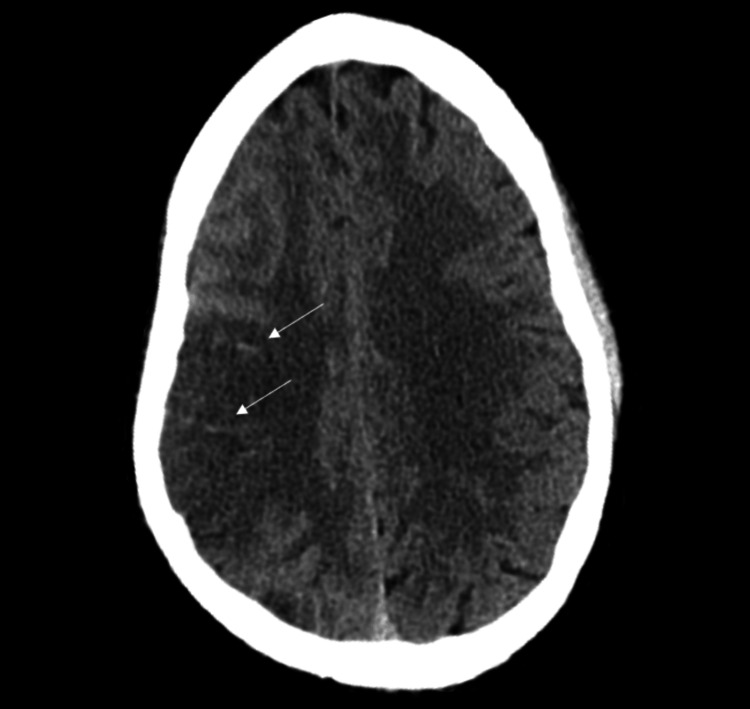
The CT of the head demonstrates right cerebral acute infarcts with gyral hyperdensities. The white arrows point to the areas of hemorrhagic transformation.
